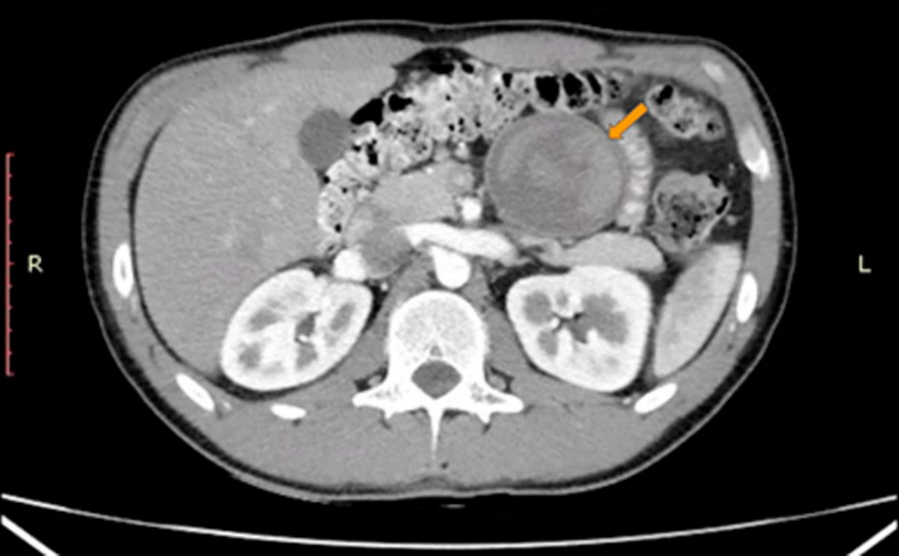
Axial section of contrast-enhanced abdominopelvic computed tomography showing a 6-cm cystic tumor with mixed density content in contact with the anterior border of the body and tail of the pancreas, small bowel, and mesenteric vessels—marked with an orange arrow
